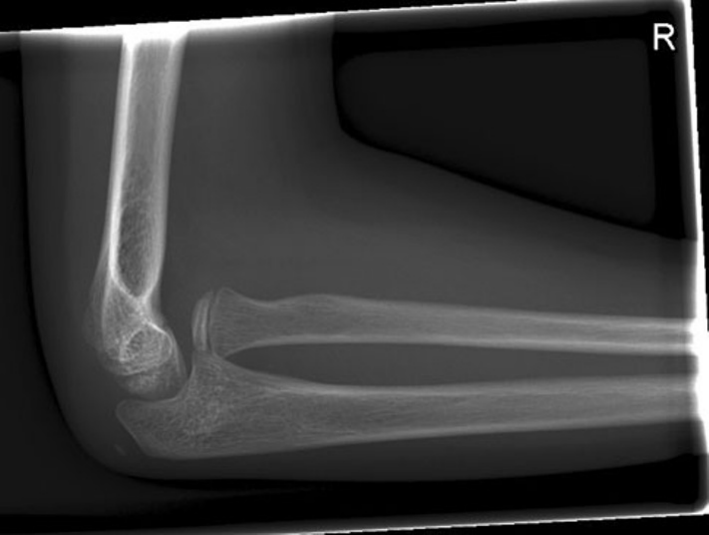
Lateral radiograph of the patients’ right elbow. Note the dislocation of the radial head
